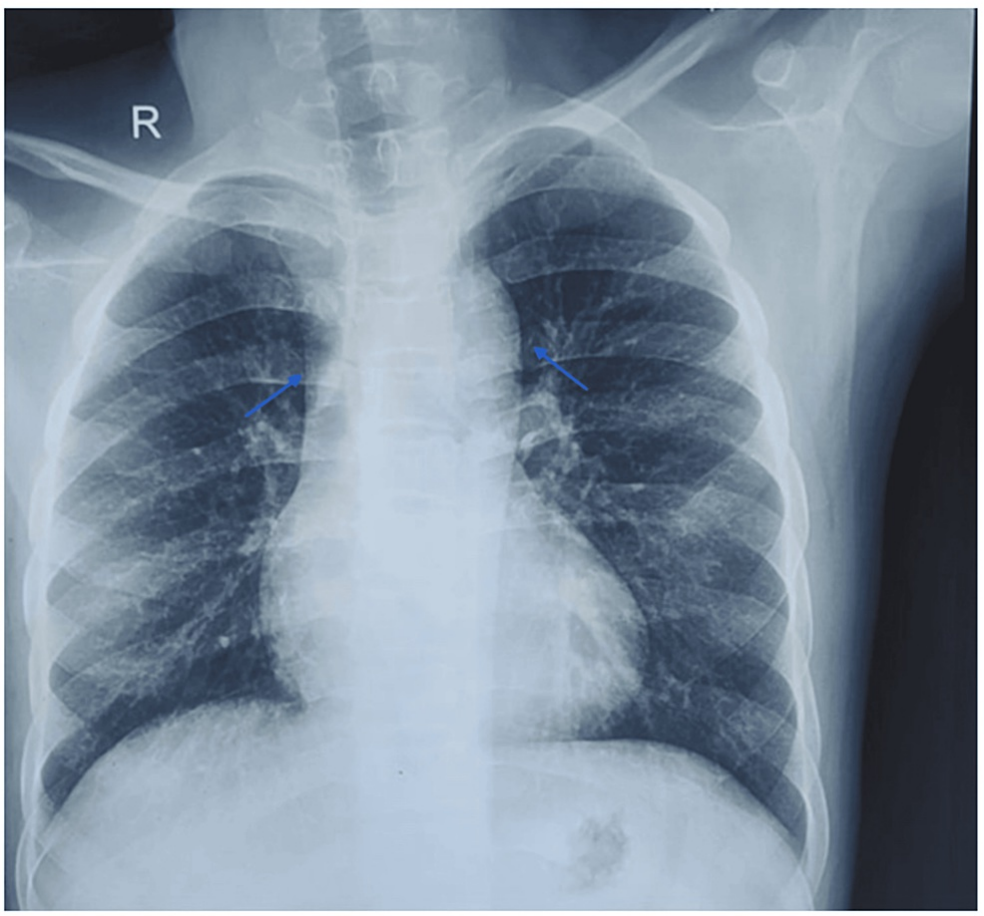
Posteroanterior view of chest radiograph revealing enlarged aorta, concave pulmonary artery, and right ventricular apex (blue arrow)
